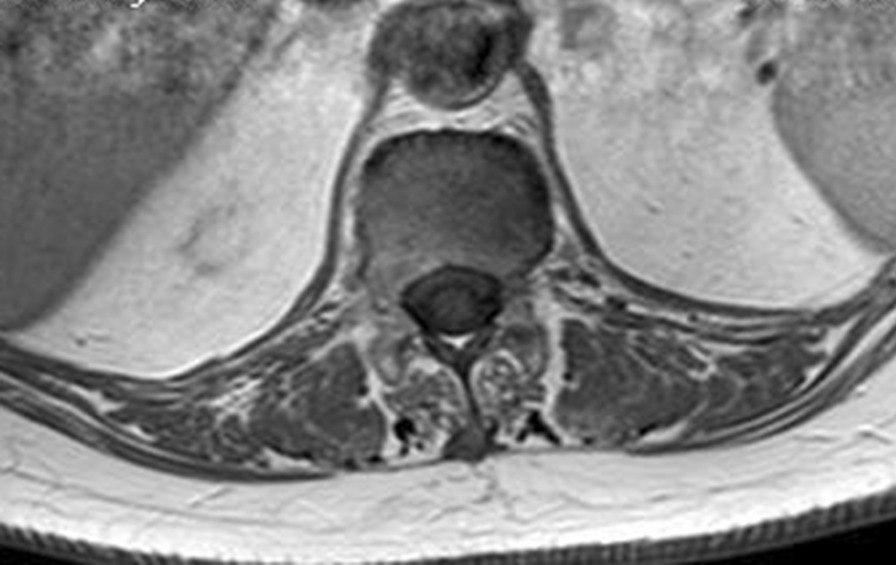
Axial T1 injected magnetic resonance imaging showing a complete resolution of the paravertebral abscess that was originally adjacent to T12
